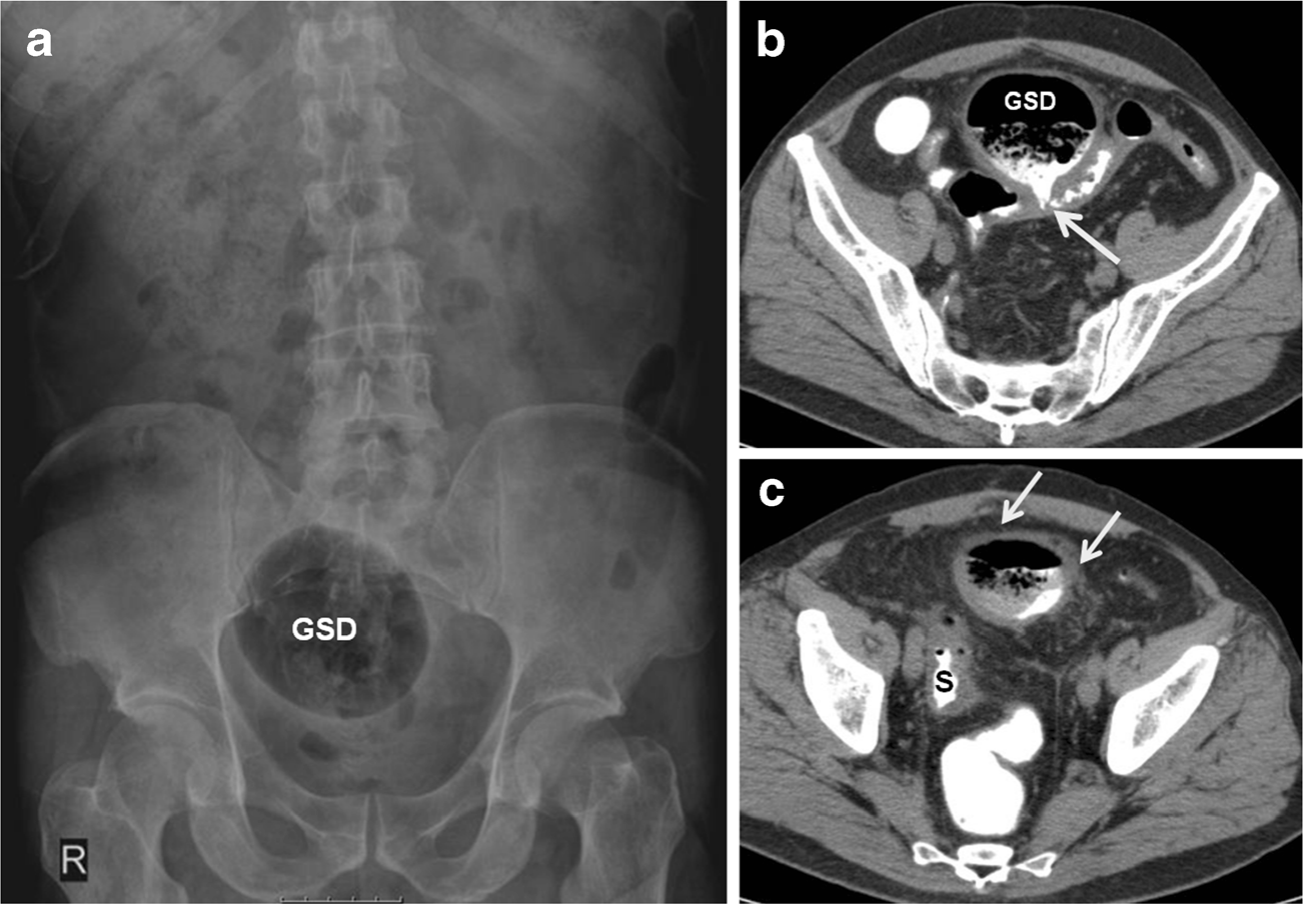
A 68-year-old man with giant sigmoid diverticulum (GSD) associated with acute diverticulitis. Abdominal radiograph (a) shows a large air-filled structure throughout the right pelvis, consistent with GSD. Axial (b and c) contrast-enhanced CT images through the pelvis show a predominantly gas-filled structure with air–contrast material level arising (arrow) from the sigmoid colon (S). The thickened wall of the diverticulum and the surrounding fat infiltration denote acute diverticulitis (arrows)
